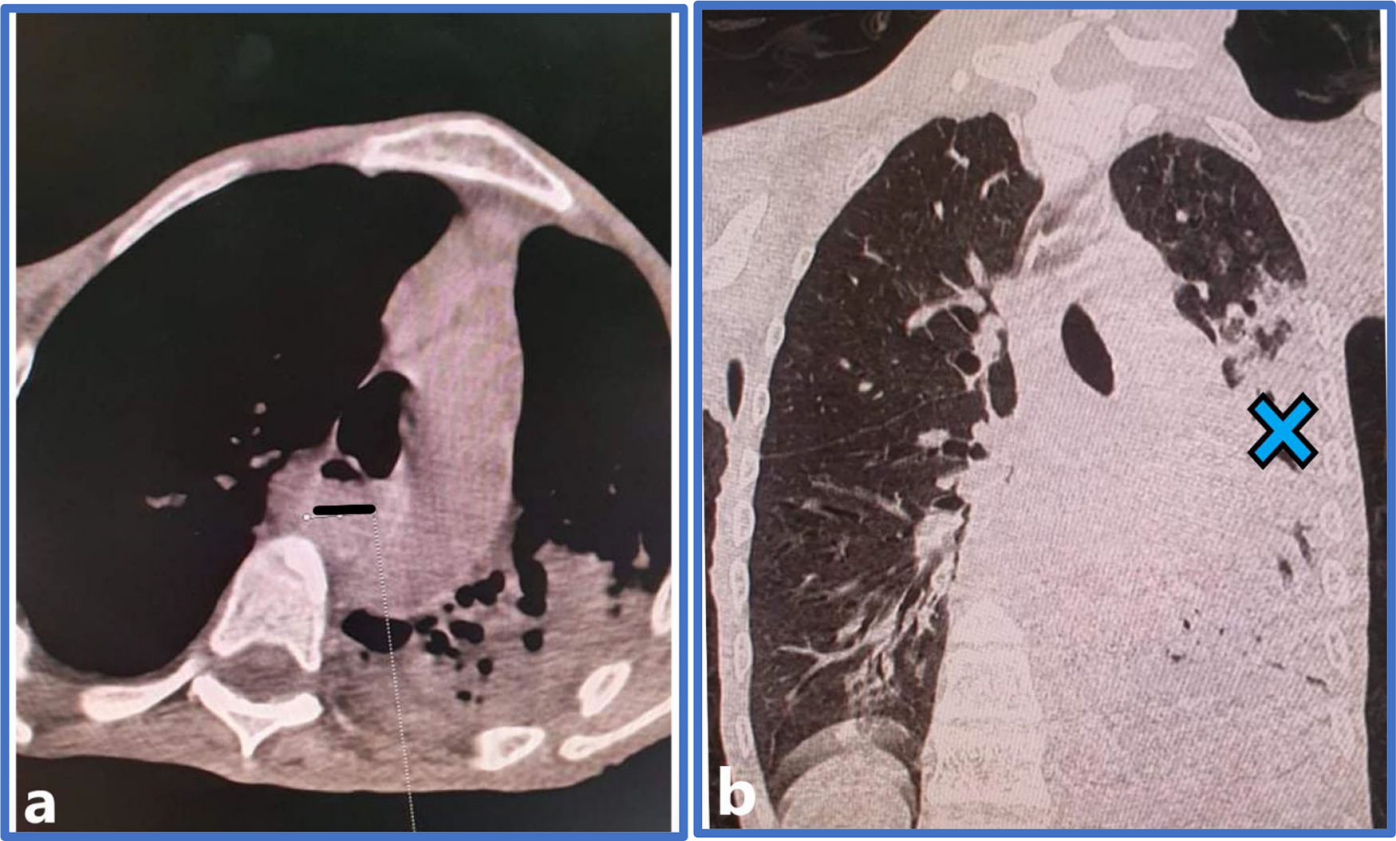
Chest computed tomography with an axial (a) and coronal (b) cuts, revealing esophageal stasis (a, Black line) and pneumonia (b, Blue cross).
